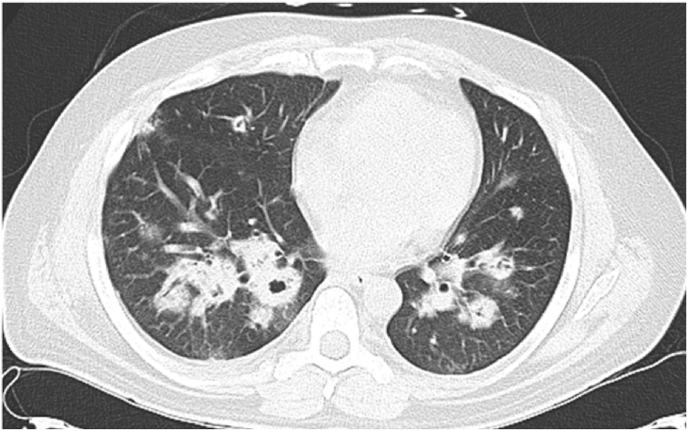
Computed Tomography Chest. CT Chest shows multiple patchy consolidations throughout both lungs, some of which are cavitating and are located primarily at the periphery.
